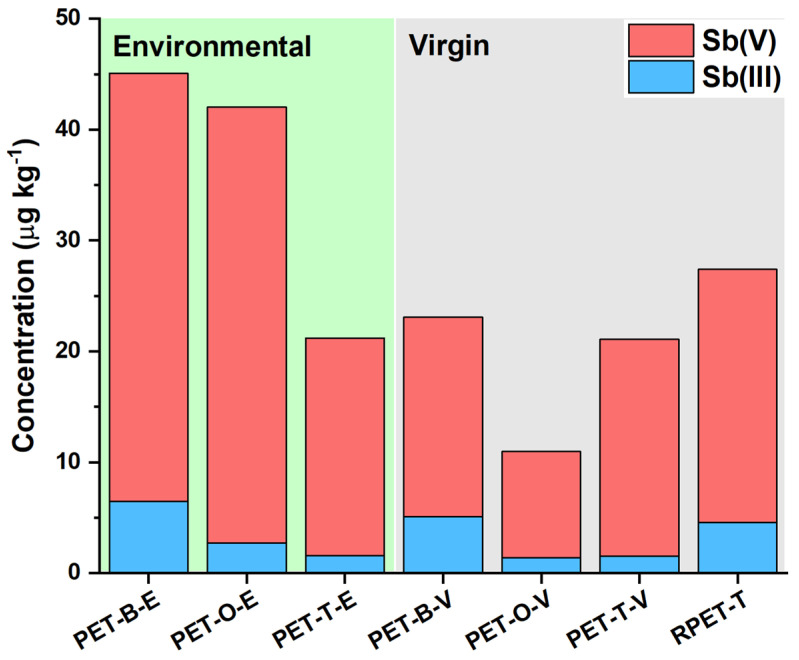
Concentration of Sb(III) and Sb(V) (μg kg^−1^ in solid sample) leached from PET samples after the ultrasound-assisted extraction in HNO_3_ 0.5 M.
